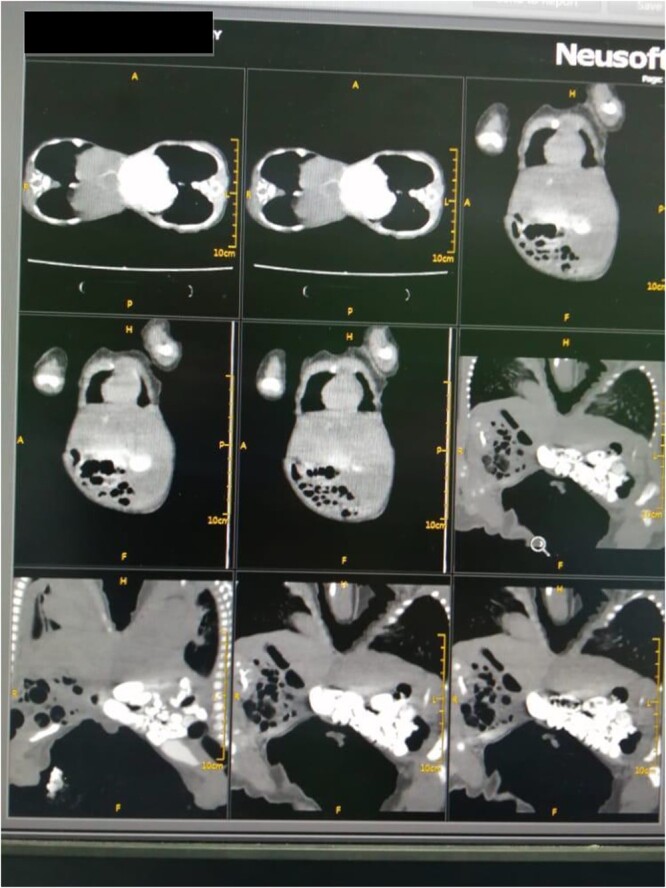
Abdominal computed tomography revealed a fusion of the liver through a large isthmus with normal liver and without vascular connections.
